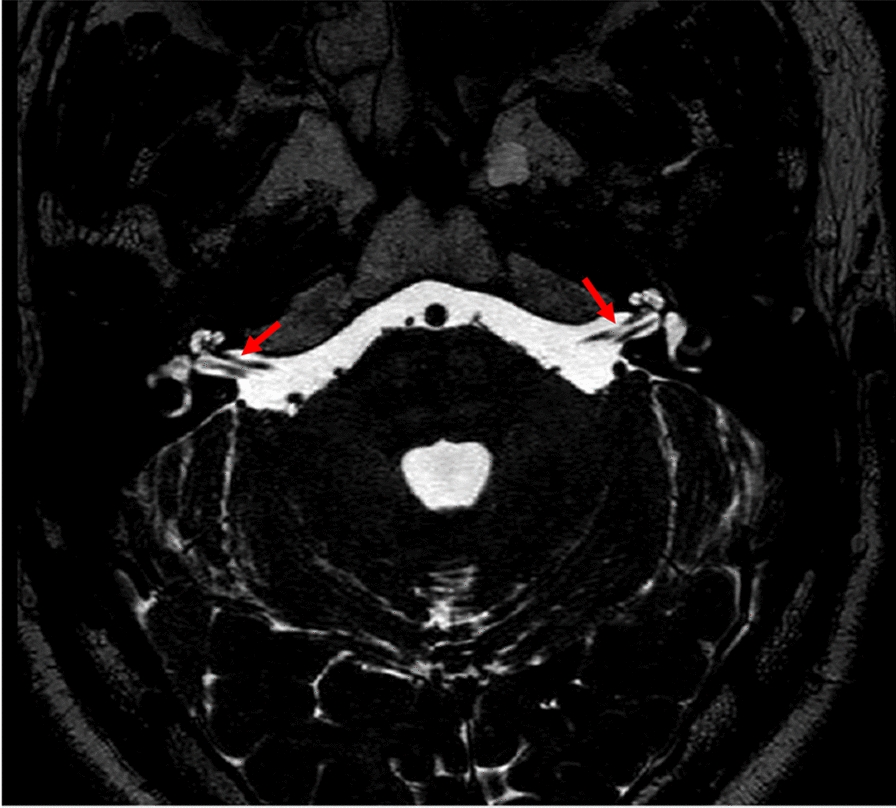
The MRI image of intracanalicular segment of the facial nerve: axial T2-weighted sequence of the IAC demonstrates the normal intracanalicular segments of the CN VII (red arrows), anterior to CN VIII
